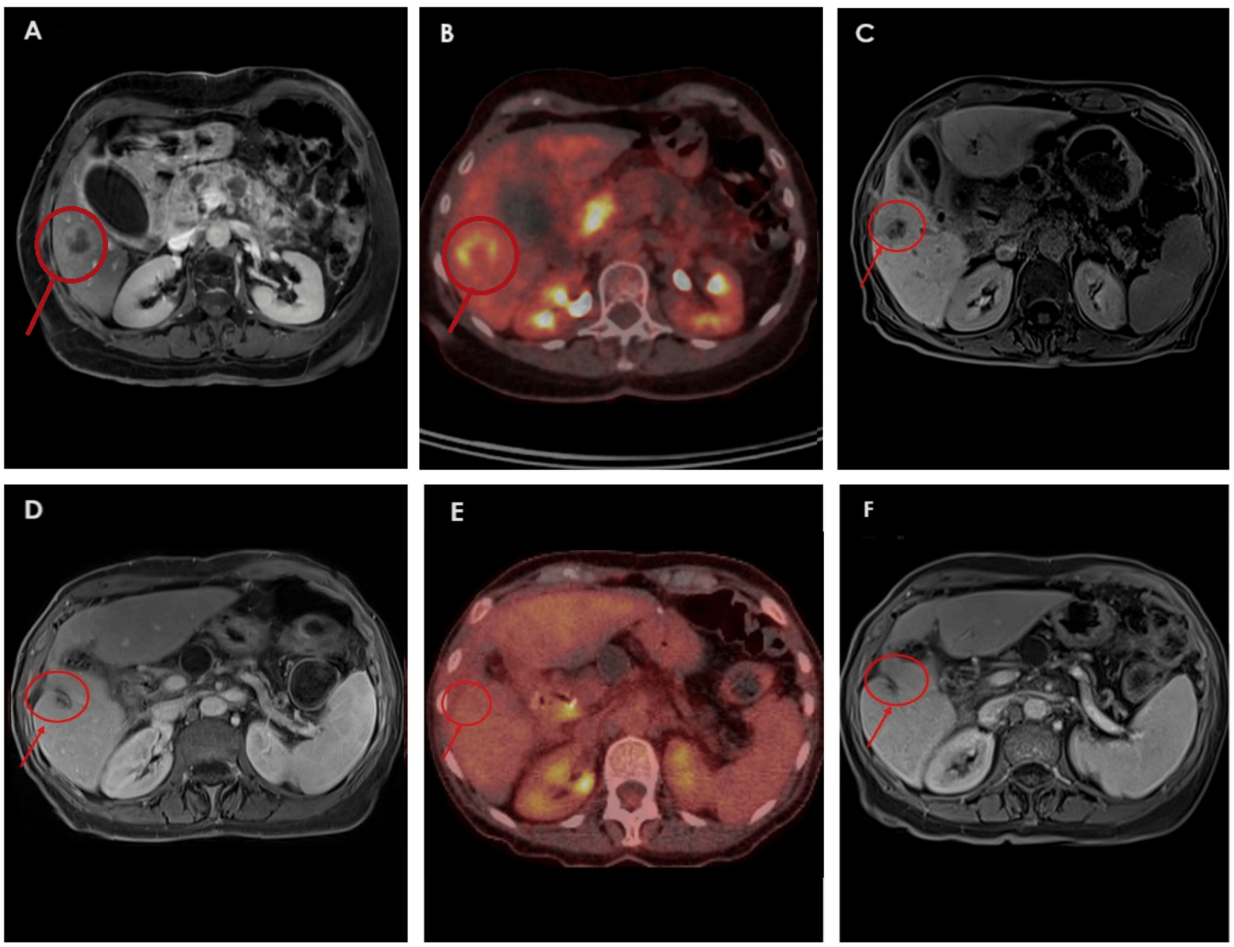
Sequential imaging of a segment V liver metastasis during systemic therapy and before and after microwave ablation (A) Axial T1-weighted CE-MRI from August 26, 2023, shows a well-defined 32 mm liver metastasis with peripheral contrast enhancement in segment V. (B) (¹⁸F)FDG PET-CT from August 29, 2023, demonstrates a hypermetabolic lesion in segment V of the liver, measuring approximately 32 mm with a necrotic center and a SUVmax of 7.1. (C) Axial T1-weighted CE-MRI from April 23, 2024, reveals a well-defined 14 mm hypointense lesion in segment V on a water-only Dixon VIBE sequence acquired 10 minutes after Primovist administration, consistent with a partially responding metastasis. (D) CE-MRI from October 21, 2024, shows a 10 mm lesion in segment V with low T1 signal intensity, no diffusion restriction, and no contrast enhancement—findings consistent with post-ablation changes and no evidence of residual tumor. (E) (¹⁸F)FDG PET-CT from October 15, 2024, shows no focal hypermetabolic activity in the area corresponding to the previously treated segment V lesion, consistent with a favorable post-ablation response. (F) CE-MRI from January 14, 2025, demonstrates a stable ablation zone with no evidence of recurrence or tumor viability, supporting a complete radiologic response to locoregional therapy. CE-MRI: contrast-enhanced magnetic resonance imaging; (¹⁸F)FDG PET-CT: 2-deoxy-2-(¹⁸F)fluoro-D-glucose positron emission tomography-computed tomography; SUVmax: maximum standardized uptake value
